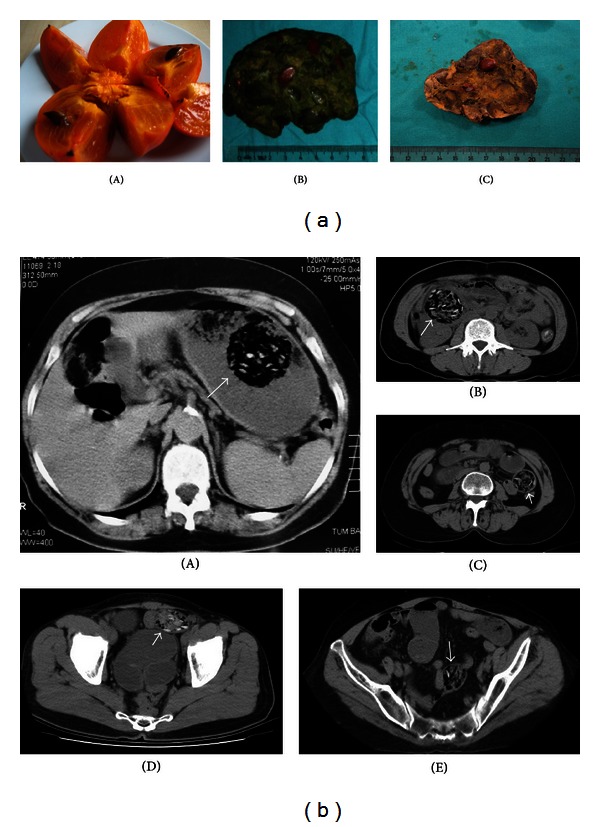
(a) Macroscopic appearance: (A) a seedy type of persimmon, (B)-(C) bezoars caused by a seedy type of persimmon. The bezoar seemed to contain a large number of seeds, and large air slits were seen. (b) CT findings of bezoars due to seedy type persimmon. Bezoar views which have different shapes and sizes and large amount of air and contained hyperdense ovoid seeds that had settled in different locations at different levels of the gastrointestinal system (arrows). (A) Stomach, (B)-(C) jejunum, and (D)-(E) ileum.
